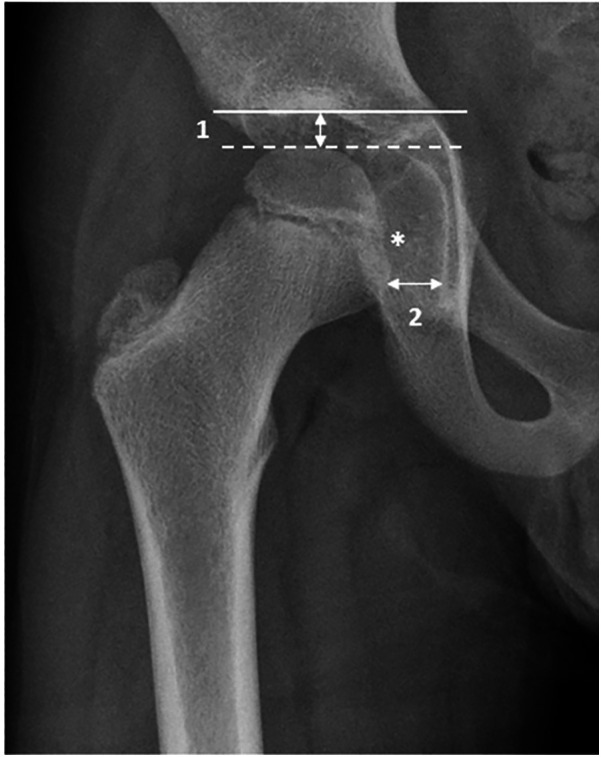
Demonstration of joint space measurement in the medial and cranial femoral head according to the study of Shelmerdine et al. (8). A solid line marks the acetabular roof, and a dashed line marks the femoral head's highest point, allowing measurement of the distance between the two lines (arrow 1). Secondly, the joint space is measured from the medial side to the femoral head's center (arrow 2) beneath the fovea (*). Reproduced with permission from “Demonstration of joint space measurements on an anteroposterior pelvic radiograph in a 9-year-old boy with juvenile idiopathic arthritis” by Susan C. Shelmerdine, Pier Luigi Di Paolo, Jasper F. M. M. Rieter, Clara Malattia, Laura Tanturri de Horatio and Karen Rosendahl, licensed under CC BY 4.0.
